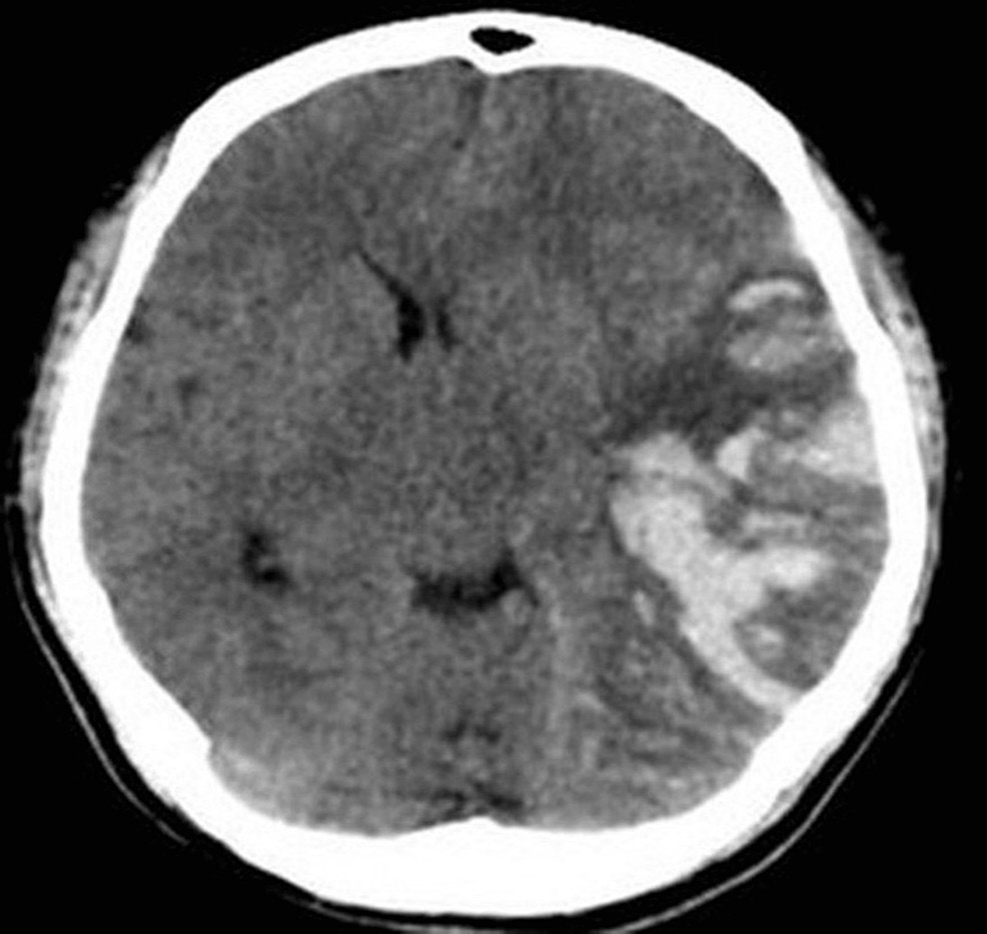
CT brain (axial view) showing acute hemorrhagic cerebral infarction at the left temporoparietal region with midline shift and mass effect.
